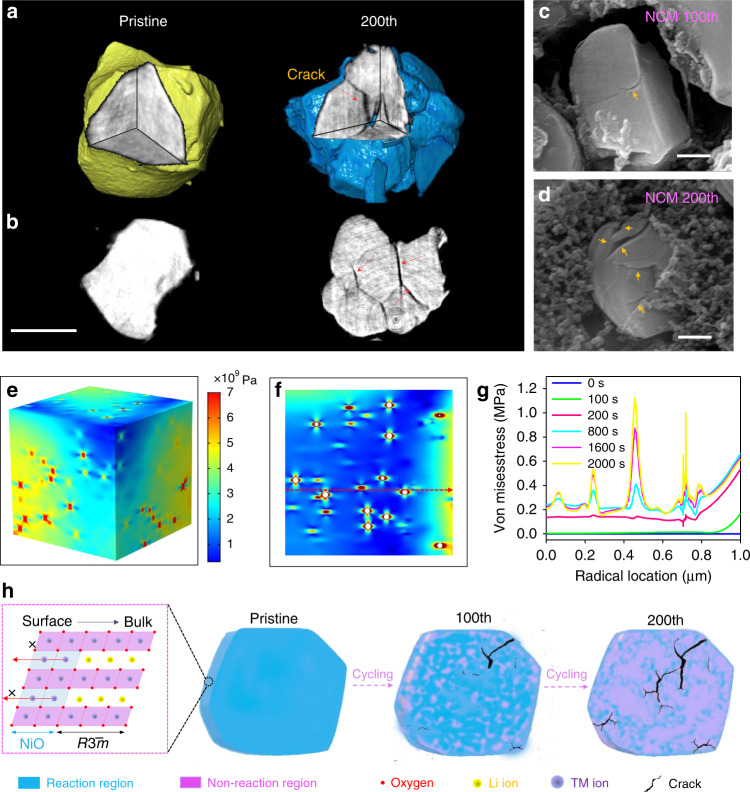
3D reconstruction and structural degradation mechanism. a, b X-ray nano-tomography reconstruction with volume rendering shows the morphological evolution of NCM after cycling. Scale bars 2 μm. c, d SEM images of the NCM microsphere after 100 and 200 cycles. Scale bars 500 nm. Equivalent stress within the NCM particles: e 3D semitransparent view. f 2D view of the cross profile. g Von Mises stress within the NCM particles along the imaginary line. h structural degradation mechanism in NCM.
